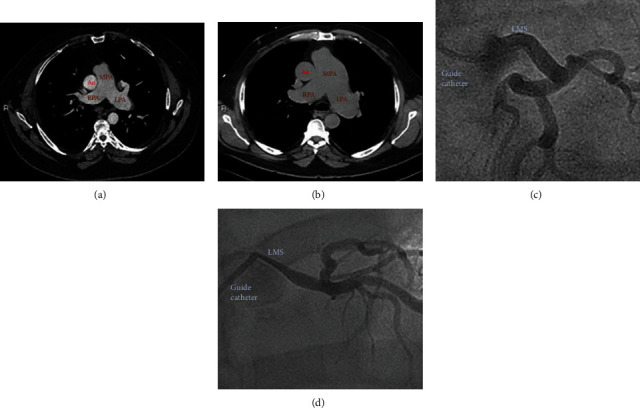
(a) CT scan showing main pulmonary artery with a diameter of 33 mm in 2013; (b) CT scan showing main pulmonary artery with a diameter of 58 mm in 2020; (c) normal appearance of LMCA in 2013; (d) severe tapering stenosis of LMCA in 2020. MPA = main pulmonary artery; RPA = right pulmonary artery; LPA = left pulmonary artery; Ao = ascending aorta; LMS = left main stem.
